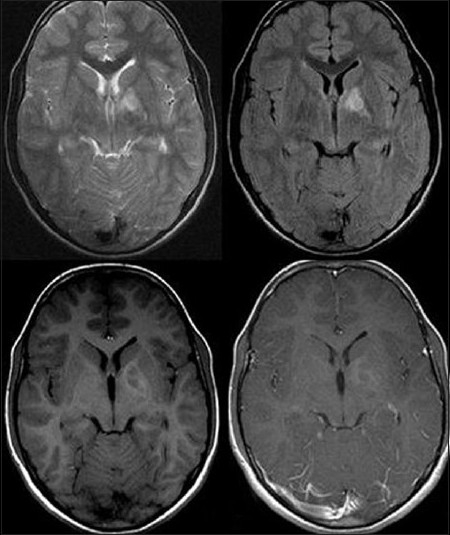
Screening magnetic resonance imaging of the brain shows a nonneoplastic hamartomatous lesion in the left basal ganglia
